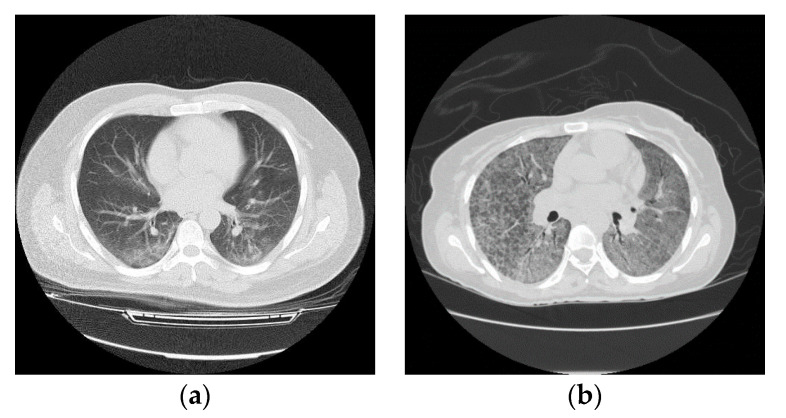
Computed tomography (CT) slices of lung in axial view: (a) infected lung with COVID-19, and (b) infected lung with pneumonia.
